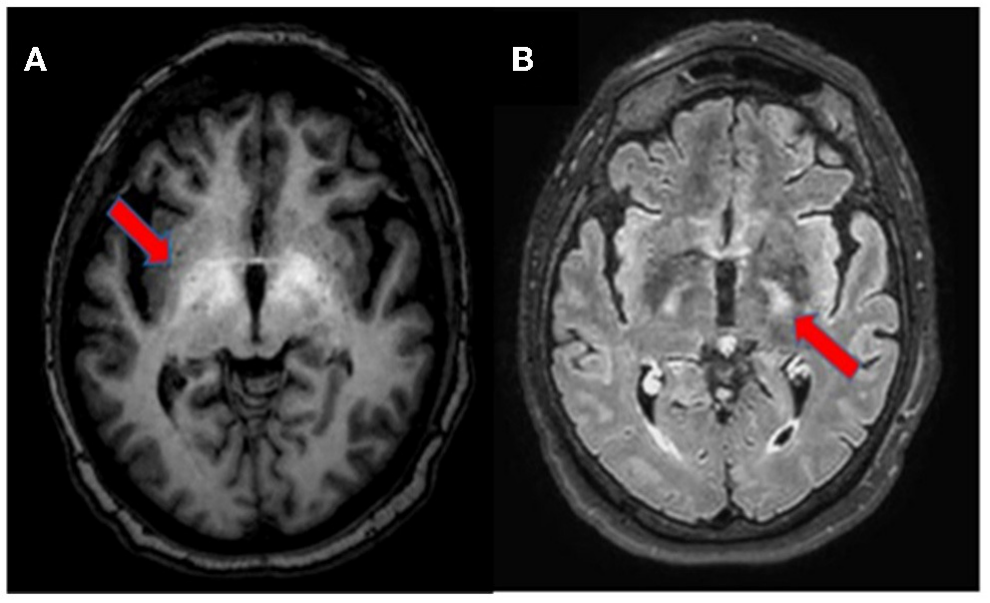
Brain MRI, axial section. T1-weighted imaging showing bilateral symmetrical hyperintensity in the globus pallidus and upper mesencephalon [(A), arrow]. T2-weighted FLAIR imaging depicting hyperintensity of the corticospinal tracts [(B), arrow].
